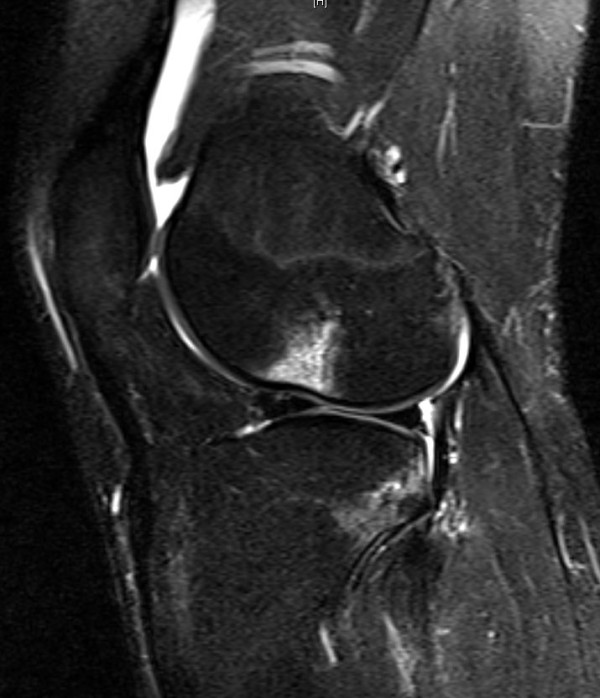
Sagittal fat-suppressed T2-weighted image shows bone contusions in the lateral femoral condyle and posterolateral tibia plateau.
